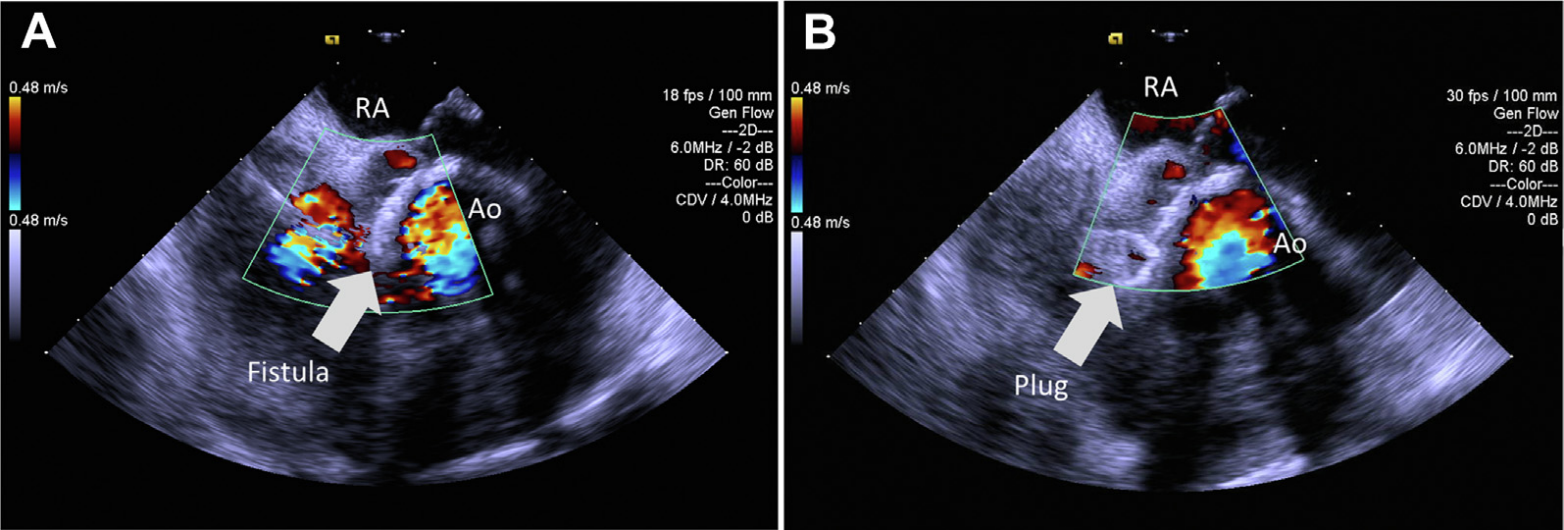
Intracardiac Echocardiography With Color Doppler Showing LVOT-to-LA Fistula Before and After Amplatzer Vascular Plug II Release (A) Before and (B) after Amplatzer Vascular Plug II release. Abbreviations as in Figure 1.
